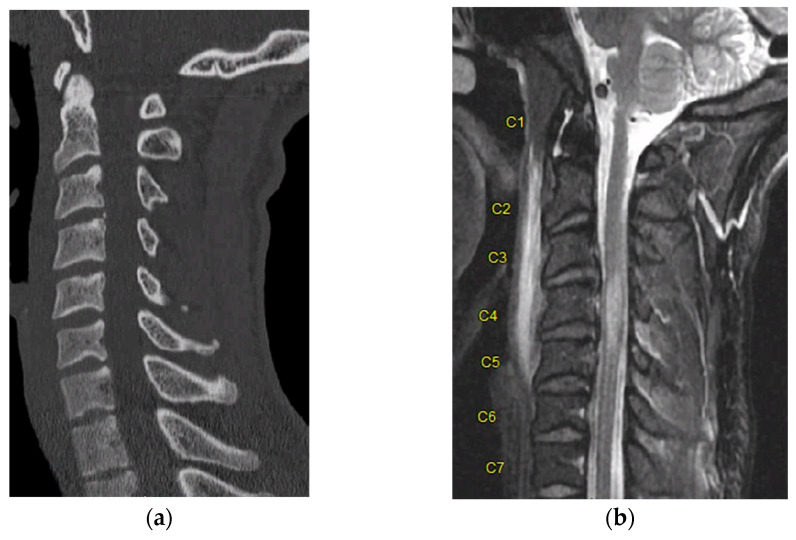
(a) Sagittal CT demonstrating no bony injury or malalignment to a trauma patient. (b) Sagittal MRI STIR sequence demonstrating cord signal change with evidence of contusion spanning from C3–C5. “C1–C7” designate each corresponding spinal level.
